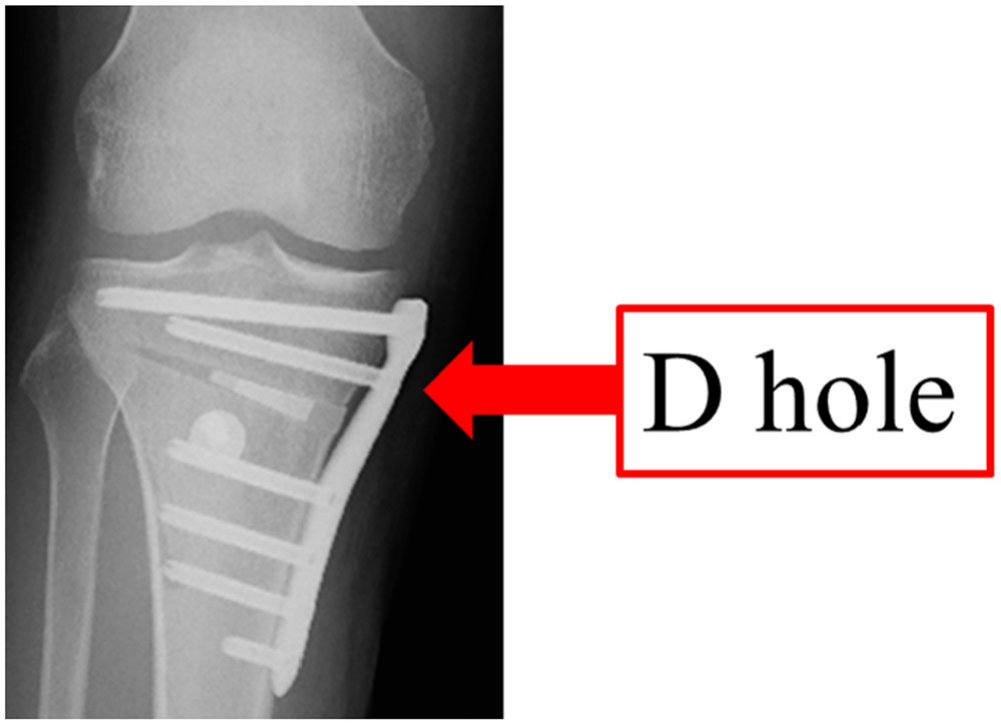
Postoperative radiography of open wedge distal tuberosity tibial osteotomy.
